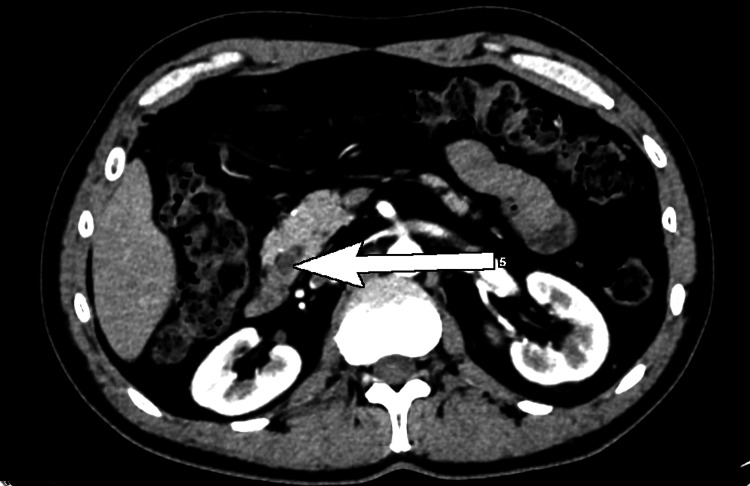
Computed tomography, axial view; a concrement with a diameter of 8.5 mm (white arrow) in the intrapancreatic part of the common bile duct.
